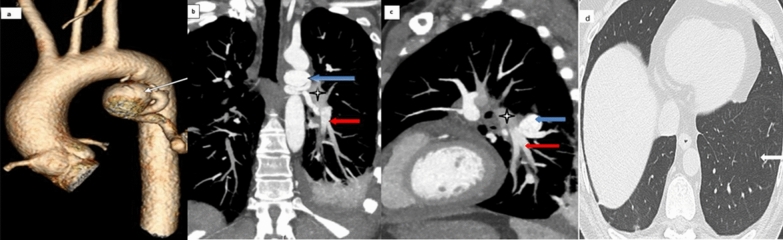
CTA demonstrating an aneurysmal systemic-to-pulmonary arterial connection with MAPCA-like features. a Coronal volume-rendered reconstruction shows a tortuous systemic vessel arising from the descending thoracic aorta with focal saccular aneurysmal dilatation (white arrow). b Coronal maximum-intensity projection image acquired in the aortic phase demonstrates the aneurysmal systemic-to-pulmonary vessel (blue arrow) extending toward the left lower lobe pulmonary arterial circulation (red arrow). Relative lack of opacification in the proximal left pulmonary artery (asterisk) is favored to reflect flow-related preferential systemic opacification rather than definitive absence. c Sagittal maximum-intensity projection image depicts the aneurysmal systemic-to-pulmonary vessel (blue arrow) and its communication with the pulmonary arterial circulation (red arrow). Relative lack of opacification in the proximal left pulmonary artery is again noted (asterisk). d Axial lung-window image demonstrates normal pulmonary parenchyma in the left lower lobe without consolidation or bronchiectasis (white arrow)
